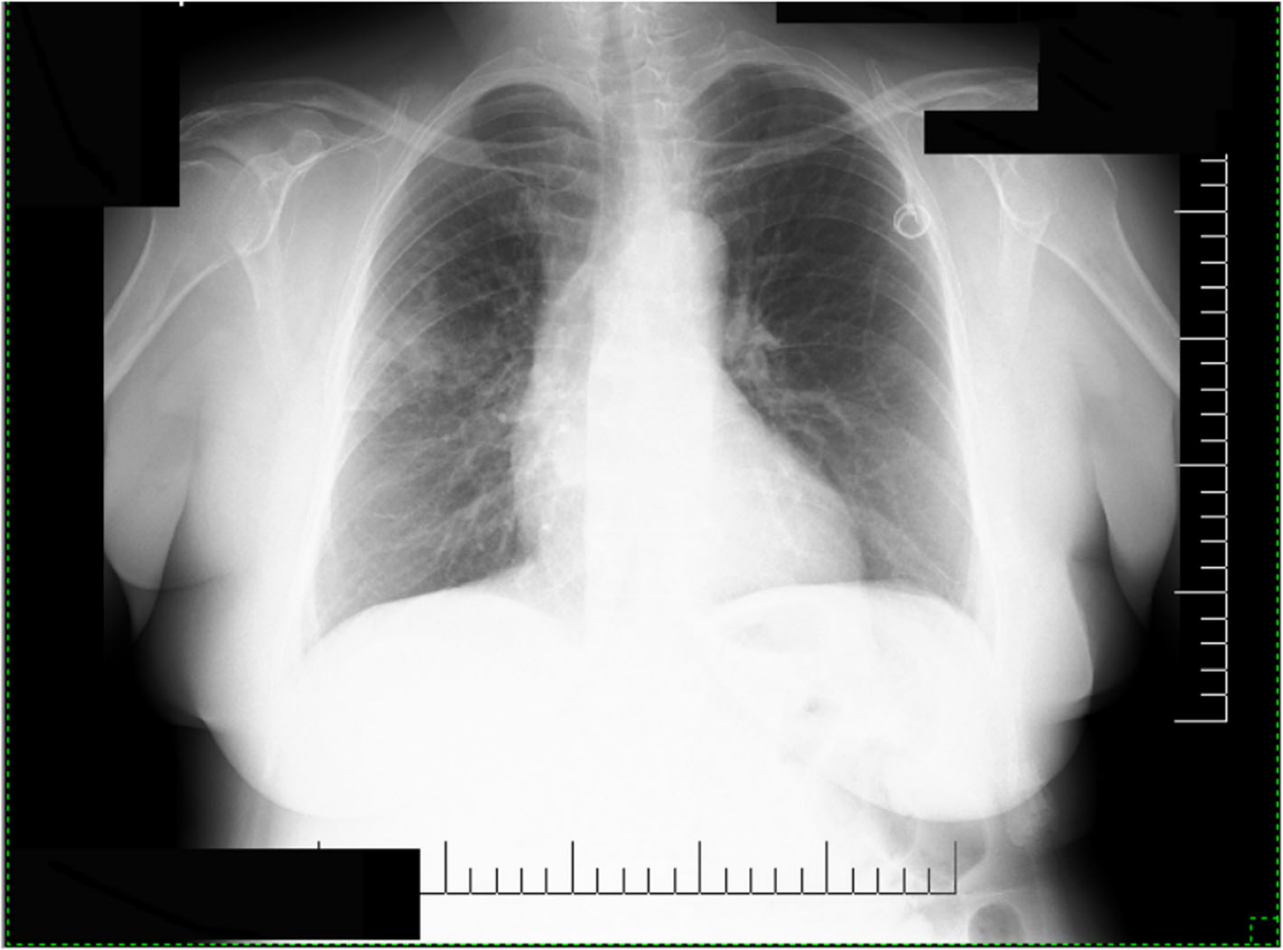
Chest radiography showed a small area of right perihilar infiltration (day 8 after chemotherapy)
